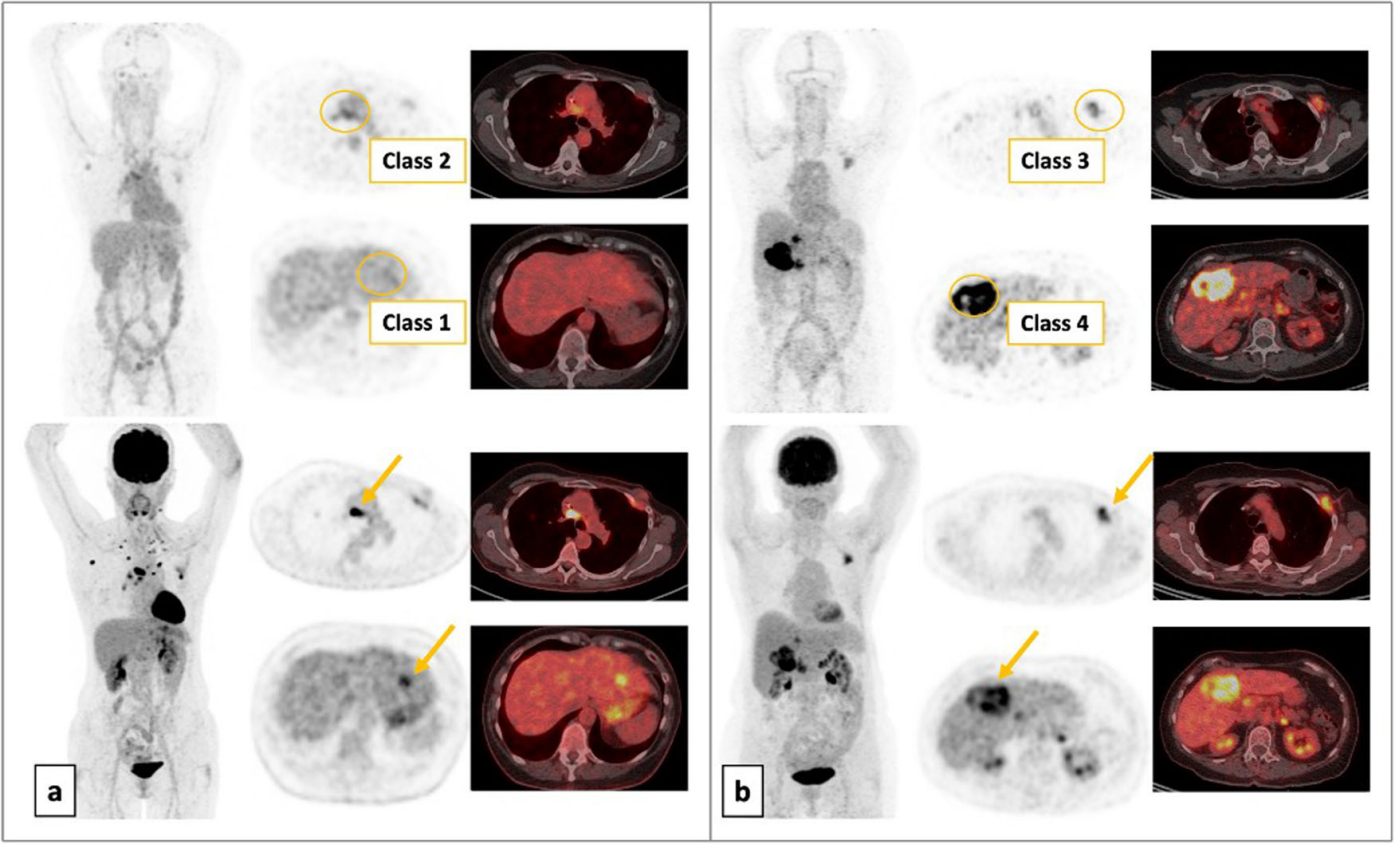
Patterns and classification of ^89^Zr-trastuzumab lesion uptake based on visual assessment, confronted with an FDG PET/CT. a HER2 PET/CT (upper row, maximum intensity projection (MIP) and axial PET and fusion images) in a patient with liver lesion showing no ^89^Zr-trastuzumab uptake (class 1, HER2-negative lesion) and a mediastinal lymph node showing uptake close to background activity (class 2, HER2-negative lesion) confronted with the FDG PET/CT images (lower row, MIP and axial PET and fusion images). b HER2 PET/CT (upper row, MIP and axial PET and fusion images) in a patient with axillary lymph node showing visible ^89^Zr-trastuzumab uptake (class 3, HER2-positive lesion) and a liver lesion showing clearly visible, high uptake (class 4, HER2-positive lesion) confronted with the FDG PET/CT images (lower row, MIP, and axial PET and fusion images).
